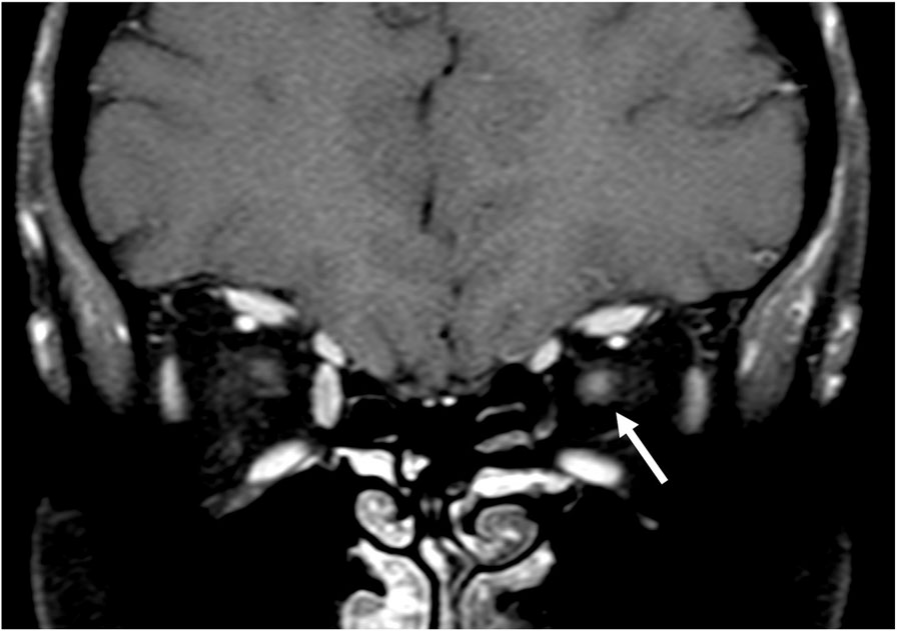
Optic neuritis. MRI T1-weighted post gadolinium fat-saturated coronal image. Note the enlargement and the enhancement of the left optic nerve (arrow)
